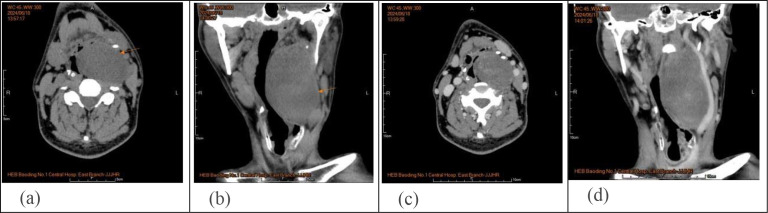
(a,b) CT of the neck: On the left side, an ovular, slightly low-density shadow, about 9.3*5.7*4.1cm in size, with a clear boundary, above the soft palate to the level of pyriform fossa, CT value of 15-39HU. Uneven density was observed between parapharyngeal and jugular vascular sheath. (c, d) Enhanced CT of the neck: The left common carotid artery, internal carotid artery and external carotid artery were compressed, and the left jugular vein was not clearly displayed.
